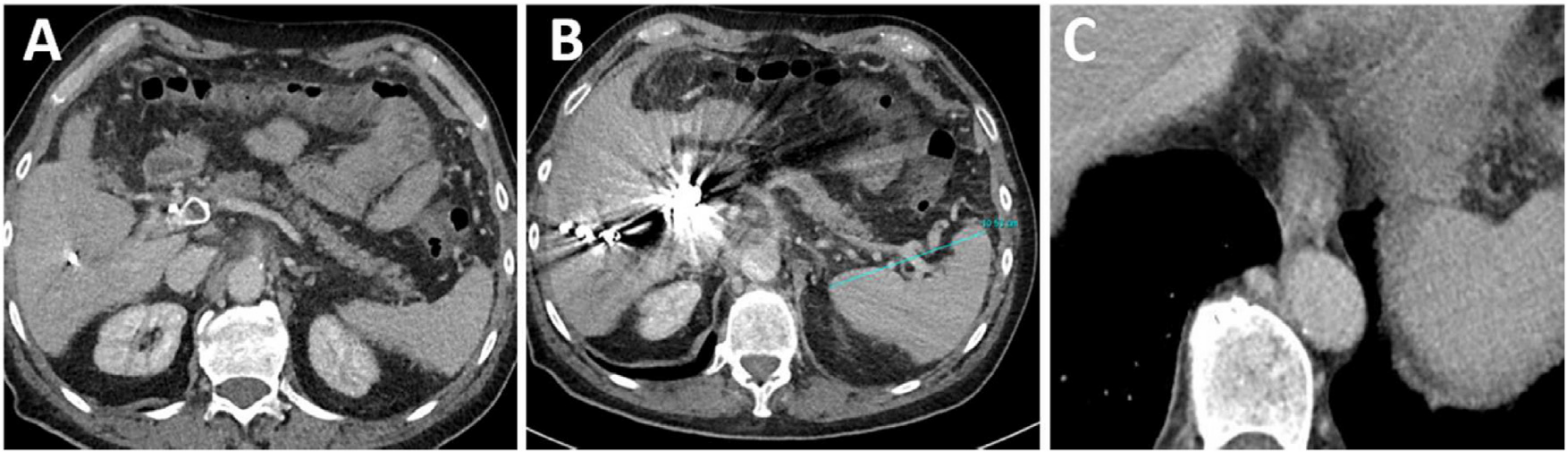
CT scan two years after the procedure. A: Intrahepatic coil and Aplatzer plug in het extrahepatic portal vein. Non-dilated splenic vein. B: Embolization materials in the liver and normal size of the spleen. C: Small esophageal varices and prominent azygos vein.
